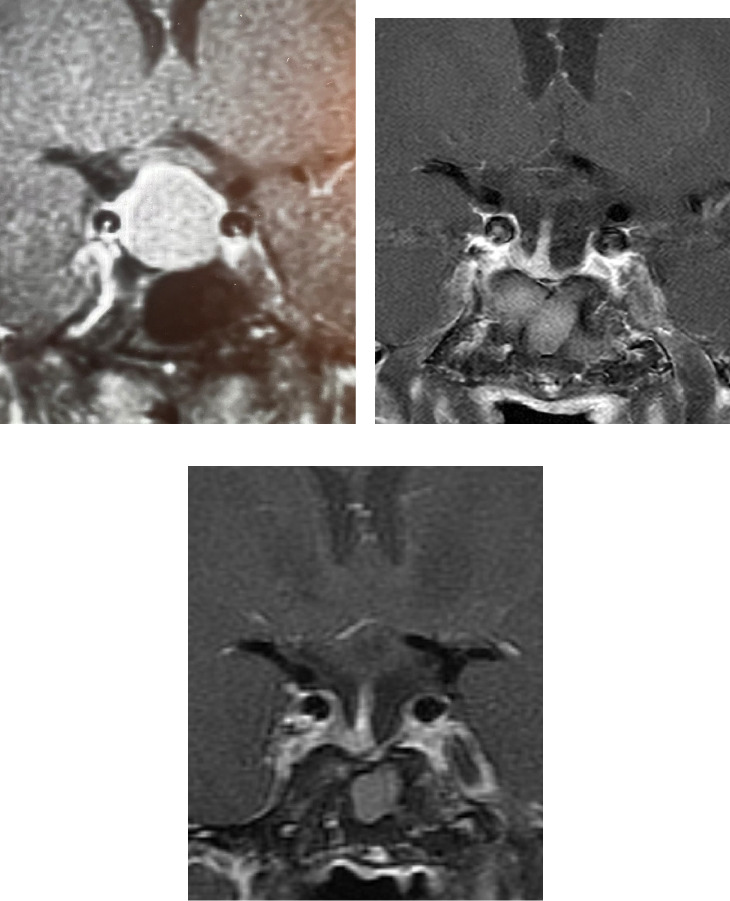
Pituitary magnetic resonance imaging. Coronal postcontrast MRIs are shown: (A) baseline prior to surgery, (B) 19 years postoperatively, and (C) 33 years postoperatively.
